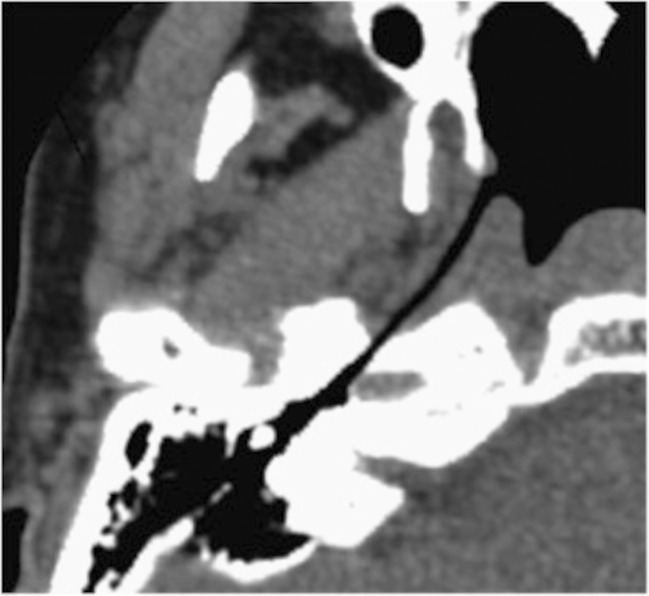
Oblique CT image of the ET and its surrounding tissues in a patient with severe symptoms of patulous ETD (reconstructed 1-mm thick, parallel and perpendicular to the long axis of the ET). The ET can be seen to be patent along its entire length. Taken from Yoshida et al. 2004 [31], with permission
